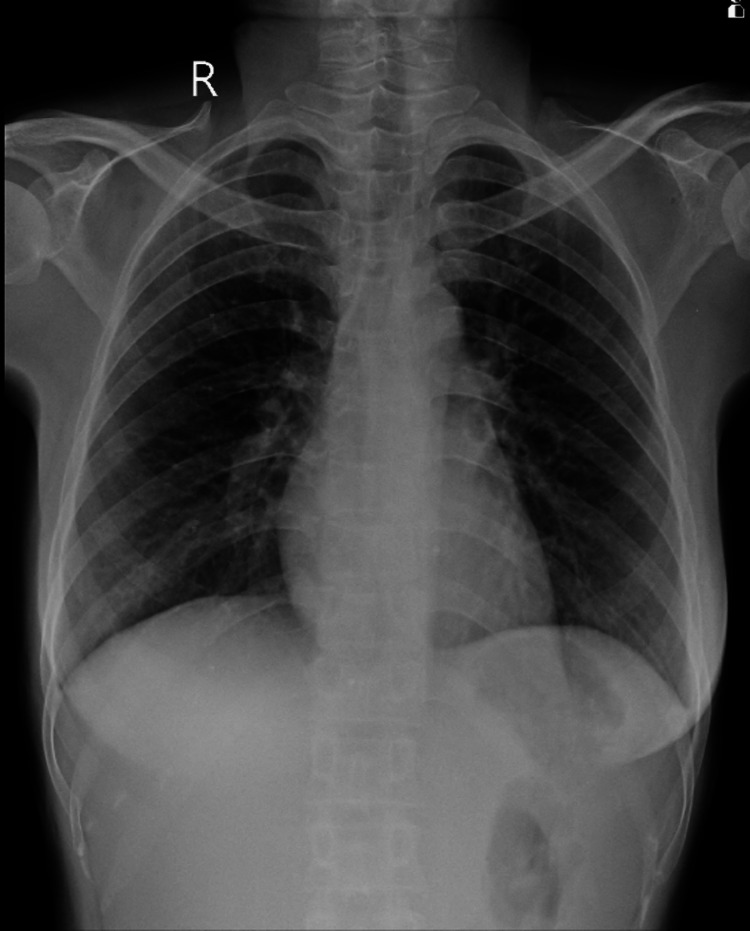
X-ray of the chest showing bilateral peripheral lower lobe opacity with increased vascular markings
